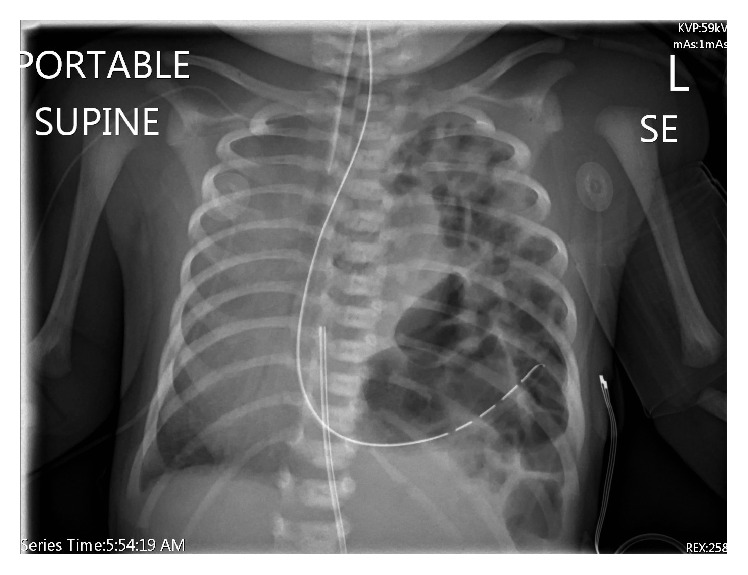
Chest radiograph showing left diaphragmatic hernia and contralateral shift of the heart and mediastinum.
